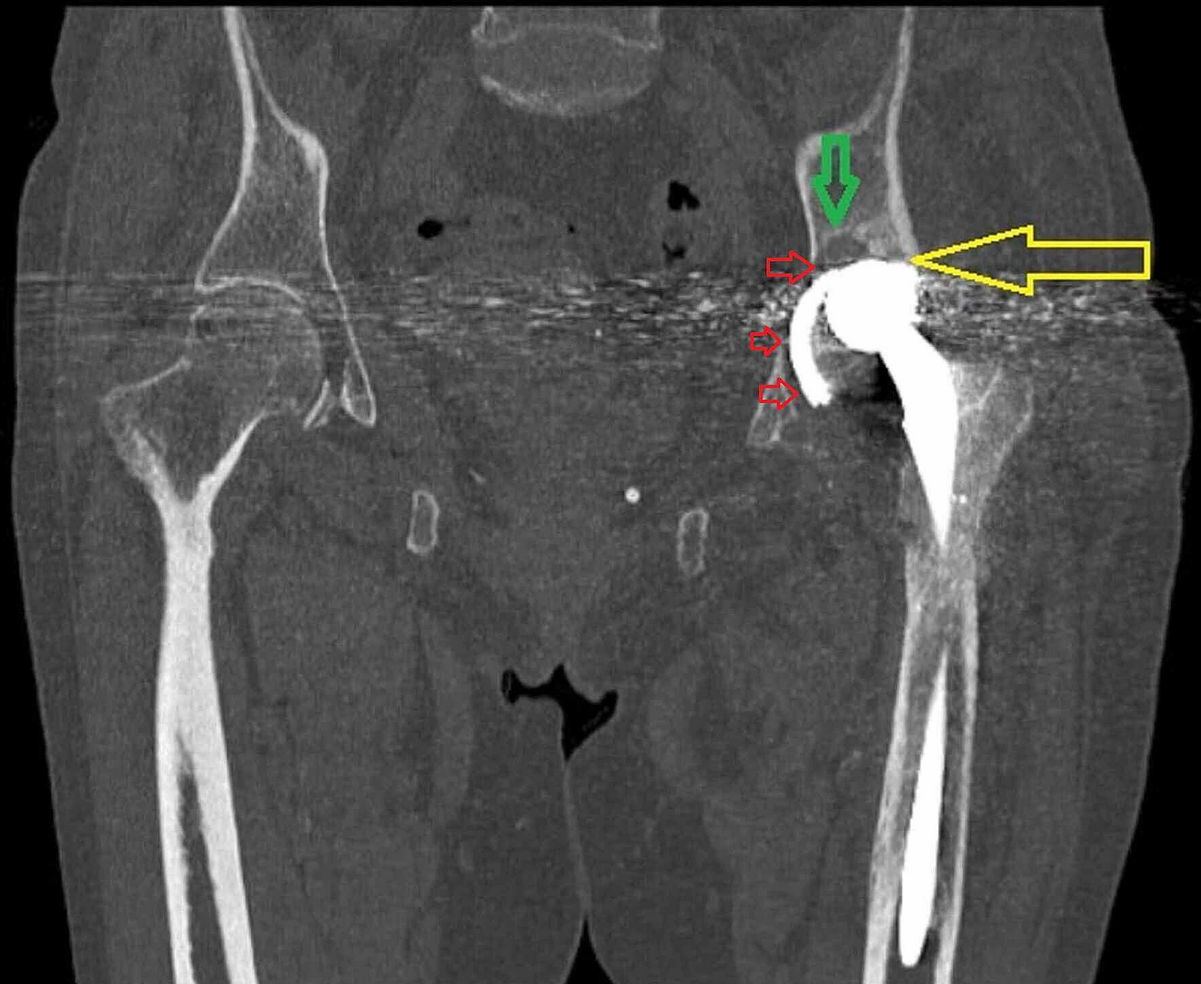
Coronal computed tomography image of the affected hip joint The yellow arrow indicates the protrusion of the femoral head through the acetabular shell; the green arrow demonstrates the focal osteolysis in Delee and Charnley zone 1, and the small red arrows indicate the radiolucent line around the acetabular component.
